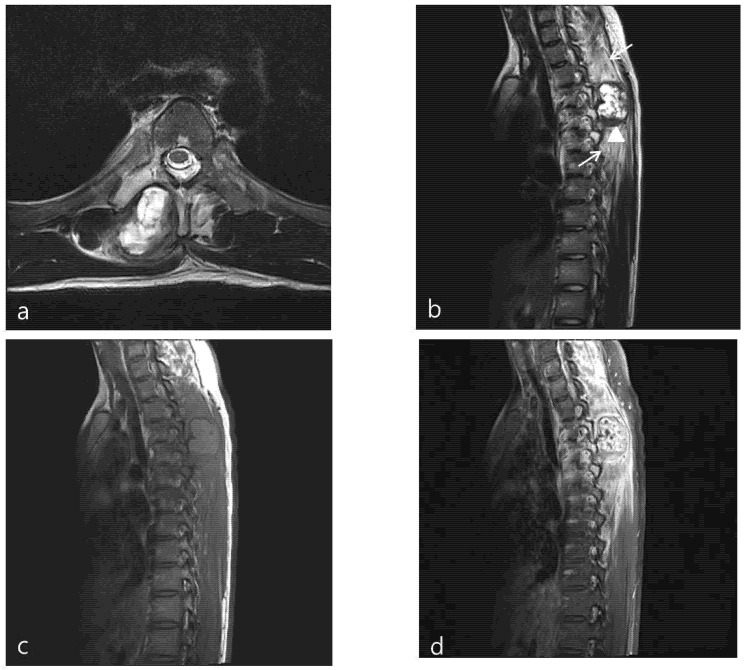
Axial (a) and sagittal (b) T2-weighted MR images show heterogeneous high SI mass in the right paraspinal area between the spinous and right transverse process of T4,5. The calcified rim (arrowhead) is shown as low SI on T2- and T1-weighted images and extensive peritumoral edema (arrows) was also noted. The mass show iso-SI on the T1-weighted image (c). On an enhanced (d) axial image, the mass shows heterogeneous enhancement.
